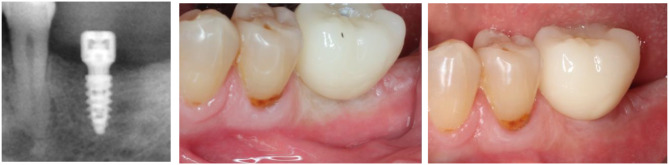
Presentation of the case. a) Radiographic control of the implant by the end of the soft tissue healing period. Note the bone grown over the shoulder of the Top DM implant, in contact with the concave shape of the transepithelial abutment. b) Blanching of the gingiva upon delivery of the implant-supported crown. Note the various blanching intensities. c) Vanished blanching that led the patient to be safely discharged
